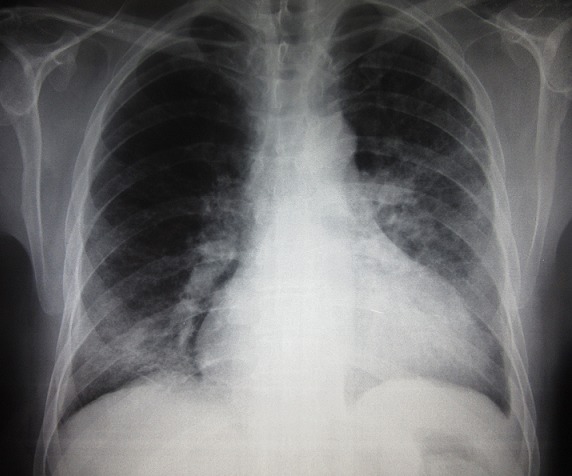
Radiographie du thorax: opacités linéaires et réticulées, micronodulaires et nodulaires diffuses, évoquant un syndrome interstitiel
